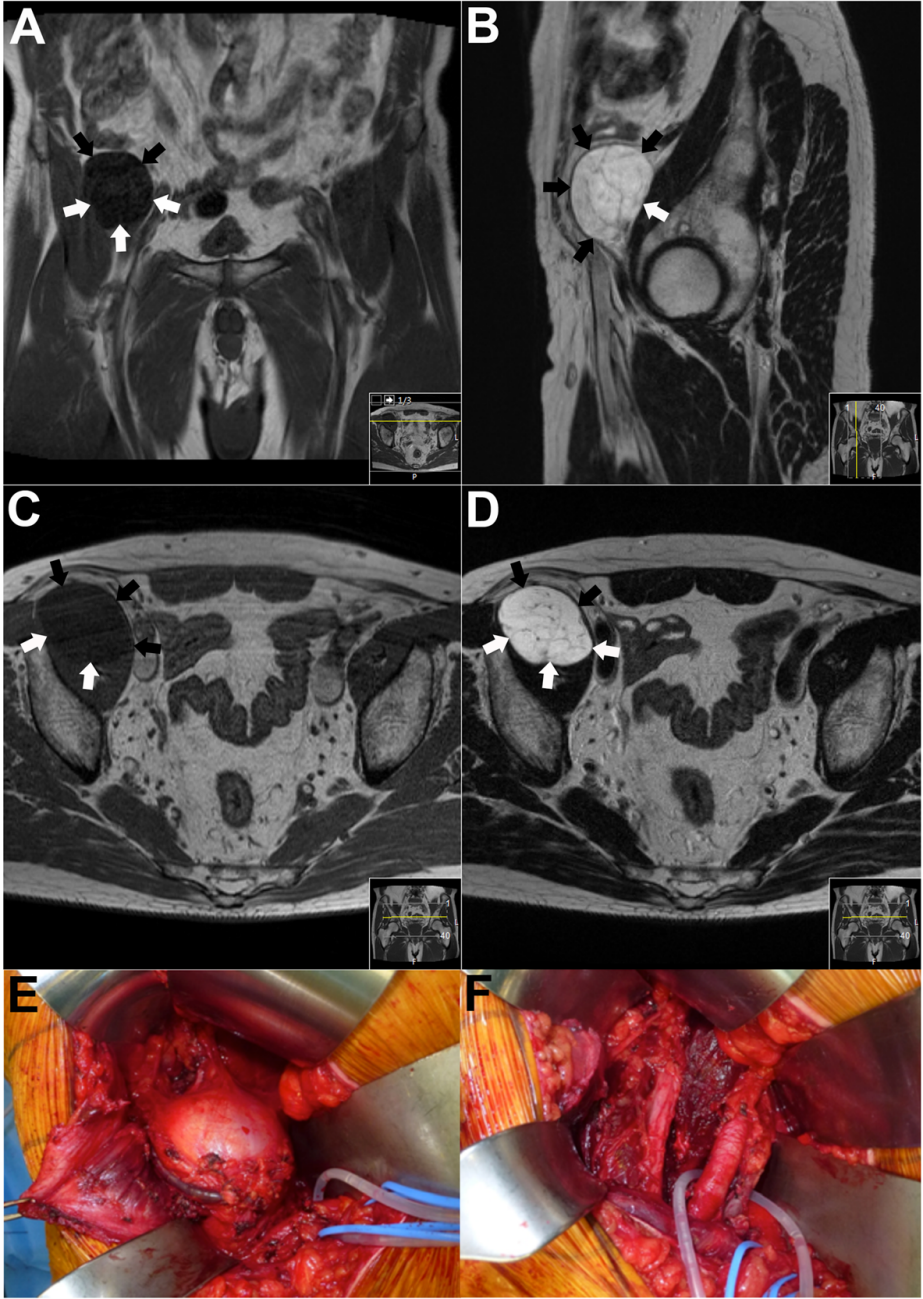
MRI imaging and intraoperative photo documentation of a 65-year-old male patient who presented with a soft tissue tumor situated in the right iliac muscle measuring approximately 5.7 × 4.8 × 4.6 cm ((a–d), tumor margins marked with arrows). The tumor was hypointens on T1 (a, c) and hyperintens on T2 (b, d) images. Core needle biopsy confirmed a low-grade (G1) myxofibrosarcoma. As the tumor was close to the external iliac vessels a marginal resection was performed. The photographs demonstrate the intraoperative situation before (e) and after (f) tumor resection; the external iliac vessels are tagged (f)
